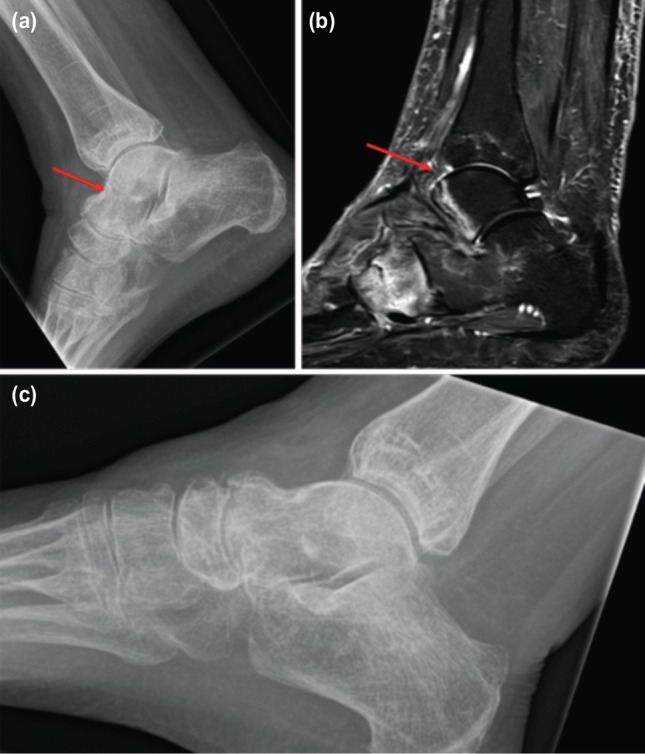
(a) Plain radiograph of the talus showing insufficiency fracture, (b) T2 weighted MRI scan of the talus showing the fracture. (c) Plain radiograph of the talus showing healed fracture.
